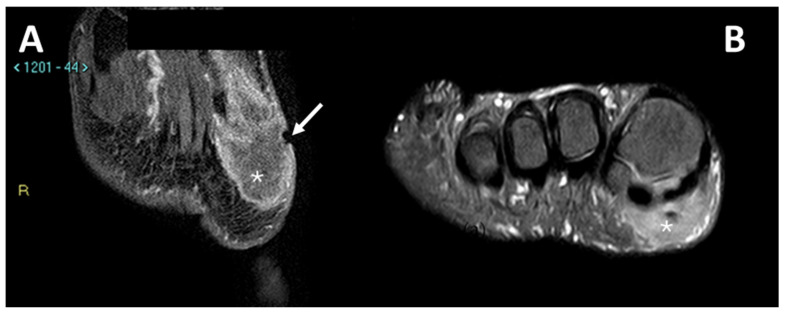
MRI in a case of CCS of the plantar region of the right foot. (A) Coronal contrast-enhanced fat-saturated T1-weighted imaging and (B) axial fat-saturated T2-weighted imaging; note the central area of macroscopic necrosis (asterisks) characterized by T2 signal hyperintensity and absence of enhancement. A slight focal infiltration in the subcutaneous tissues can be noted as well (arrow).
